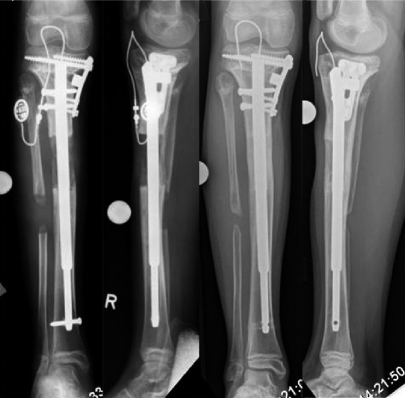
Anteroposterior and lateral radiographs 3 months (left) and 9 months (right) after surgery.
